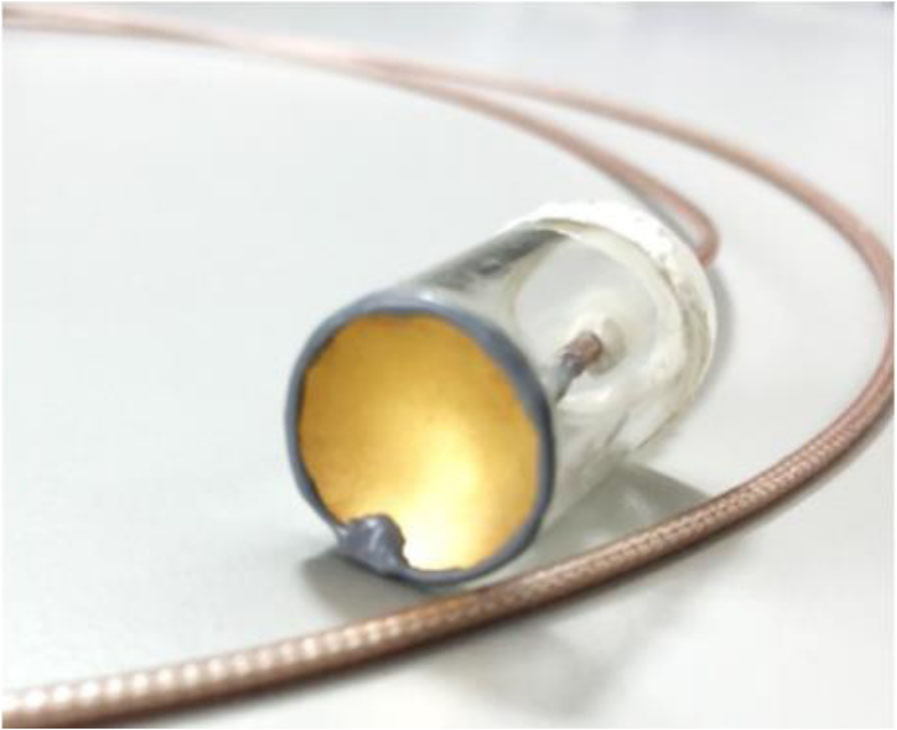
The photo of the 5­MHz focused ultrasonic transducer
